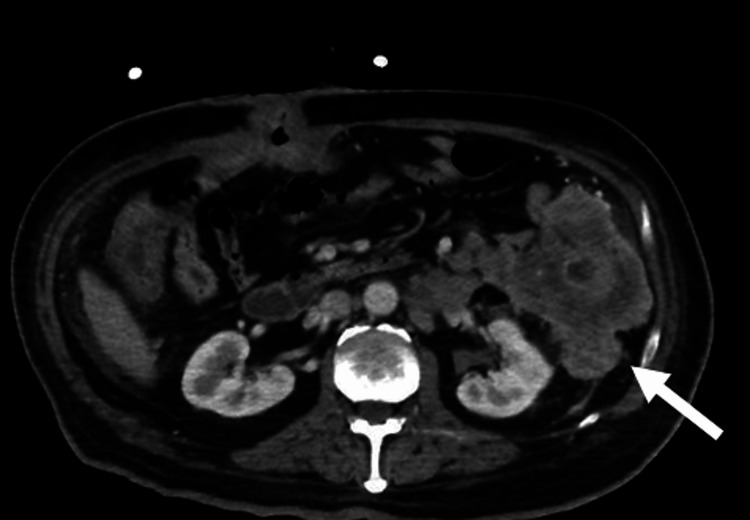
Preoperative CT abdomen and pelvis showing the outpouching of the posterior descending colon, which is consistent with the tumor invading through the colonic wall.
